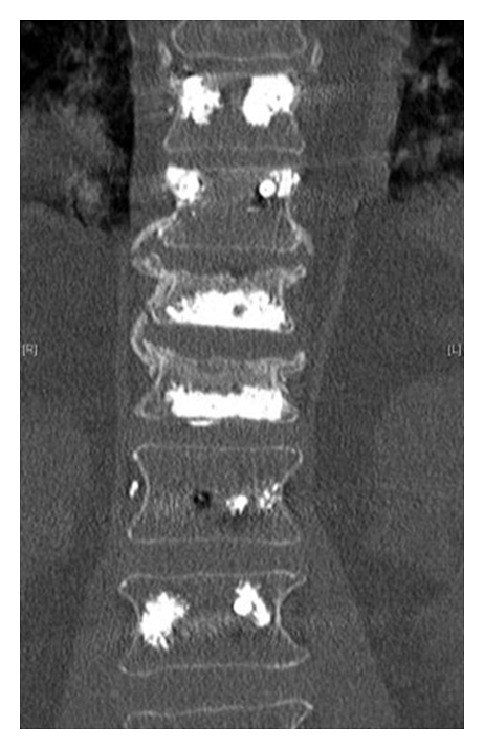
Postoperative coronal CT scan (same patient in Figure 1) showing cemented screw 2 levels above and below the fractured levels and 2 balloon kyphoplasty.
